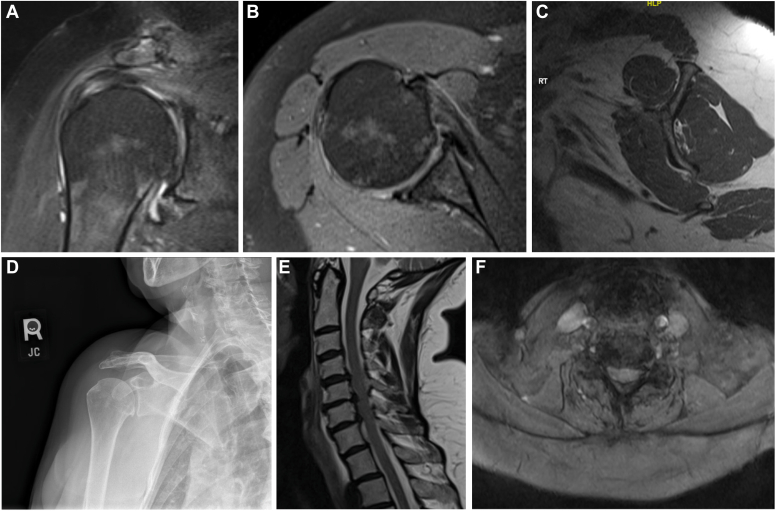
(A-D) Imaging from clinical vignette #2. (E and F) MRI from clinical vignette #2. *MRI*, magnetic resonance imaging.
